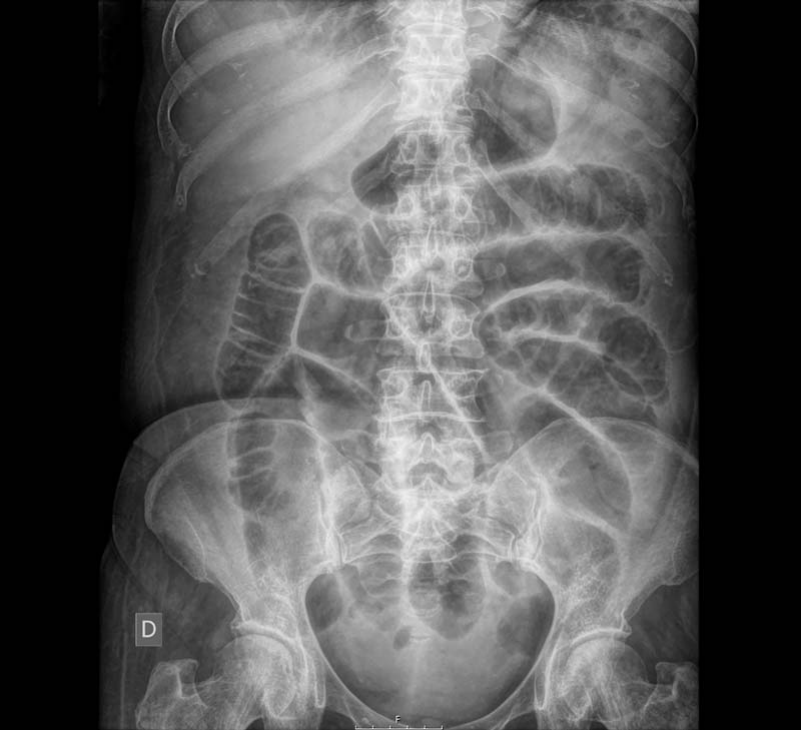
Abdominal X-ray: generalized dilatation of the small intestine without distal air in the colon or the rectum.
